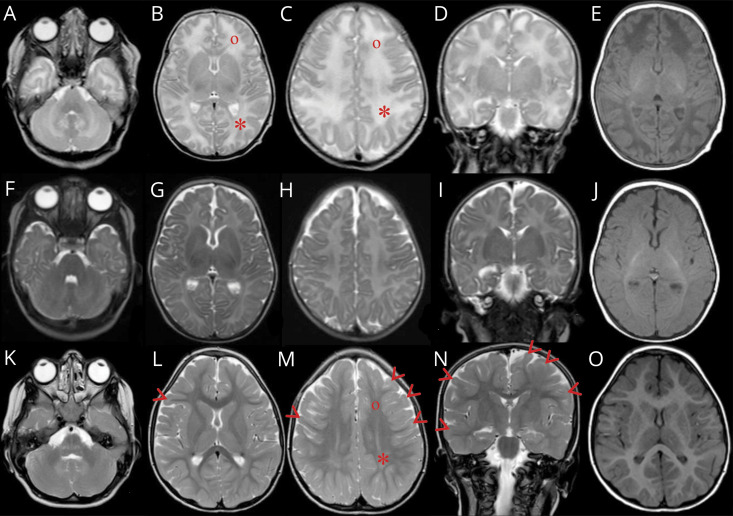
MRI of the index patient (II:3) T2-weighted MRI at ages 23 days (A–D), 2 months (F–I), and 4 years (K–N) and T1 at corresponding age (E, J, and O). Peripheral white matter in the parietal (*) compared with frontal (°) lobes shows increased signal at 23 days (B and C) that persists in parietal lobes at 4 years (M). At age 4 years, subcortical frontal and temporal white matter (arrow heads) (L–N) shows persistant high signal for age when it should have resolved by age 3 years.
